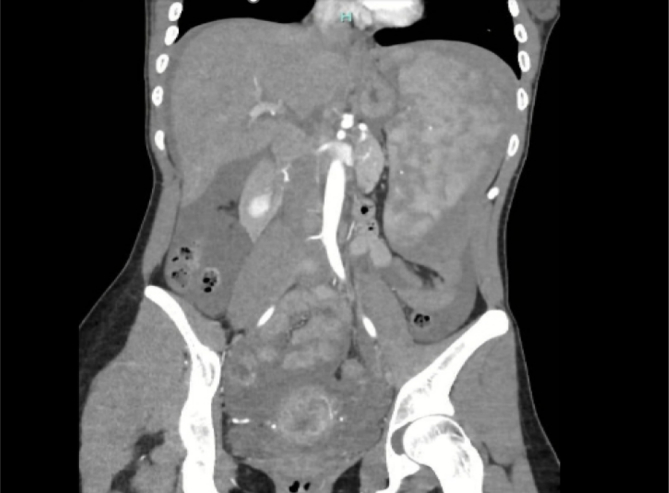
Abdominal CT-scan (coronal view).
